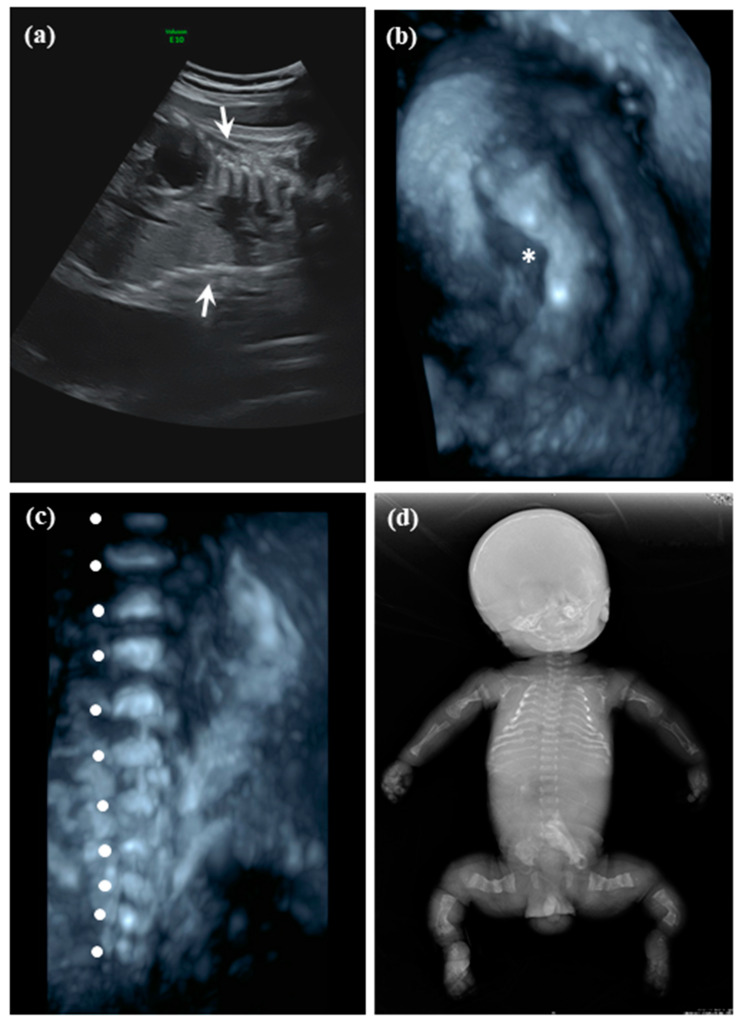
Prenatal ultrasonography of Patient 2 (a male fetus) with OI IX in Family 2 at GA = 34 weeks and 1 day showed (a) small and collapsed thoracic cage (arrow), (b) bowing of femoral bone (star), and (c) platyspondyly of spine (filled circle). Postmortem whole-body x-film showed (d) ribbon like ribs, multiple bowing and fractures of general gracile bones, and pseudoarthrosis of long bones.
